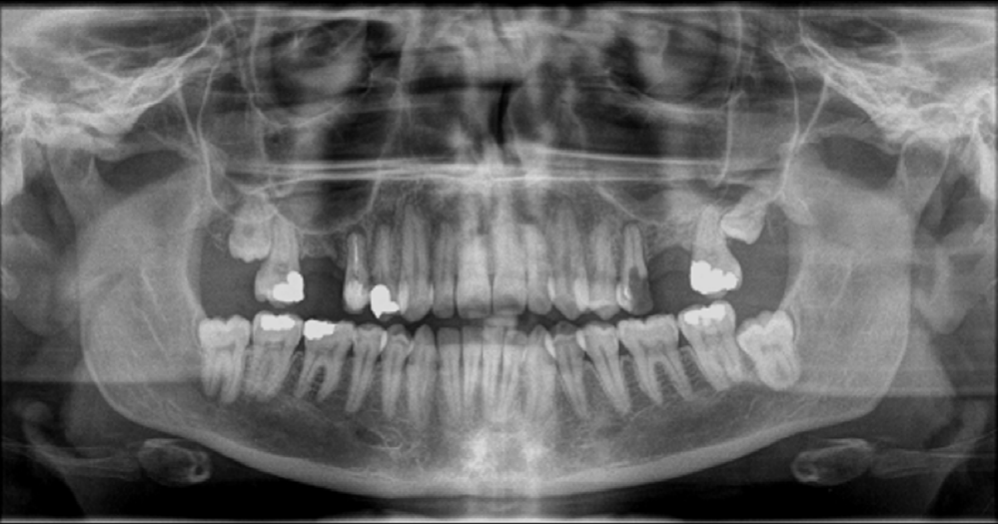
Standard orthopantomogram (OPG). It is usually acquired when a patient presents with symptoms related to upper or lower jaw or near by structures.
